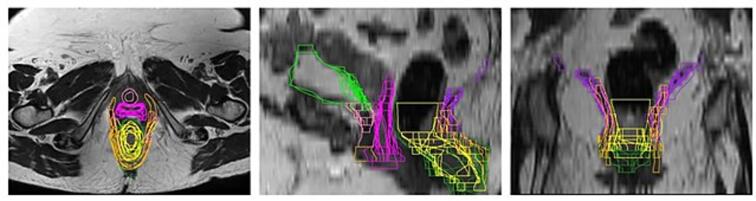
A graphical representation of interobserver variability among centres of all substructures and emerging OARs on axial (a), sagittal (b), and coronal (c) planes of T2-weighted sequence: Levator ani muscle (LAM, fuchsia); Puborectalis muscle (PRM, orange); Internal anal sphincter (IAS, yellow); External anal sphincter (IAS, forest green); Bladder base and trigone (green); Bladder neck (pink); Vagina (violet).
